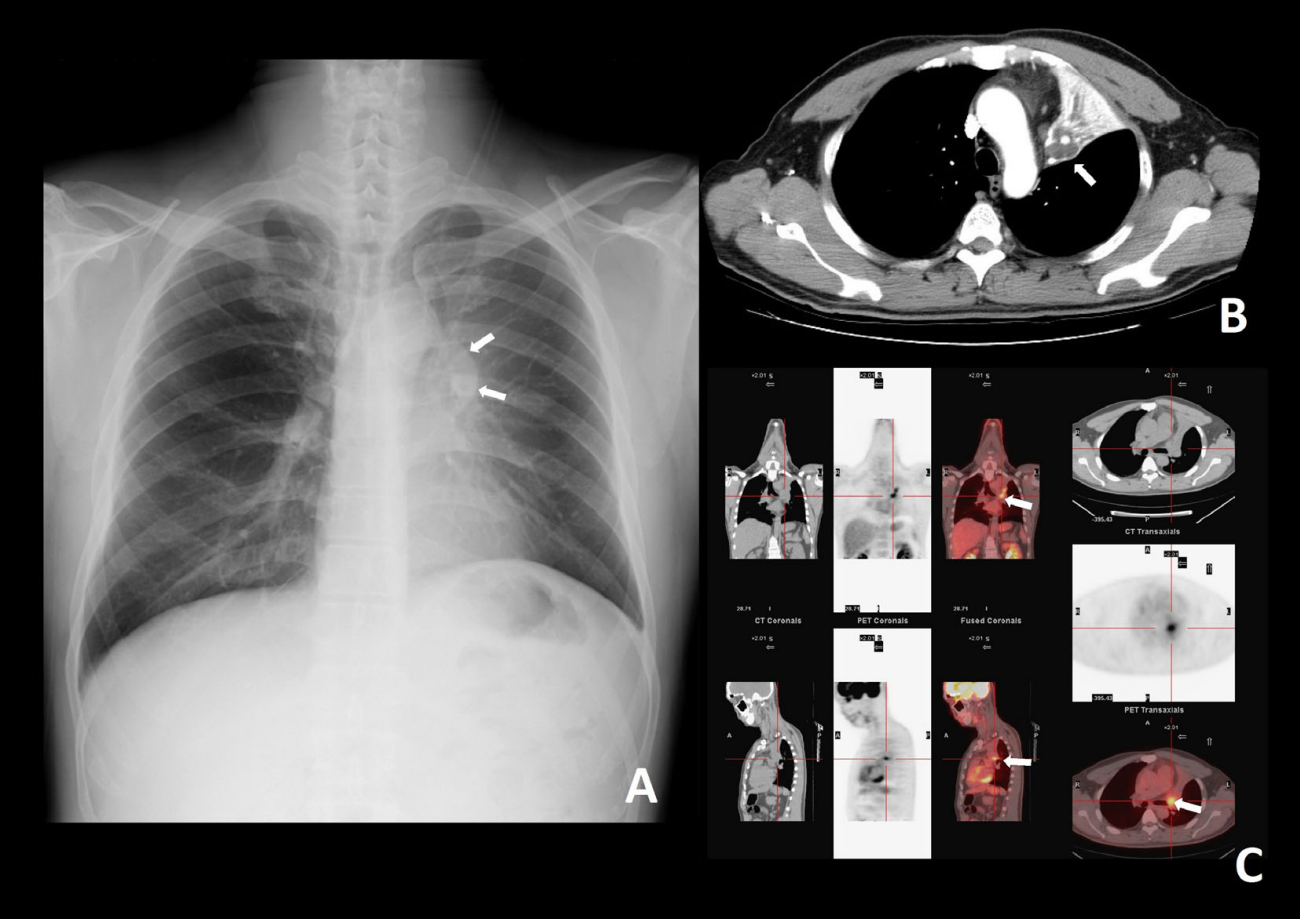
(A) Chest plain film. A protruding mass shadow is seen in the left hilar region. The shadow of the left bronchus stops at the mass. Costodiaphragmatic angles are clear. There is increased density over the left lung field with elevation of the left side of the diaphragm. These findings are indicative of a hilar mass obstructing the bronchus with collapse of the left upper lobe of lung. (B) Contrast computed tomography (CT) image, distal part of the tumor. The distal bronchus is dilated and filled with secretions. The margin between the lung parenchyma and tumor is indistinct. (C) Positron emission tomography (PET) and CT, proximal part of the tumor. An endobronchial tumor with high tracer uptake and clear margins is visible.
